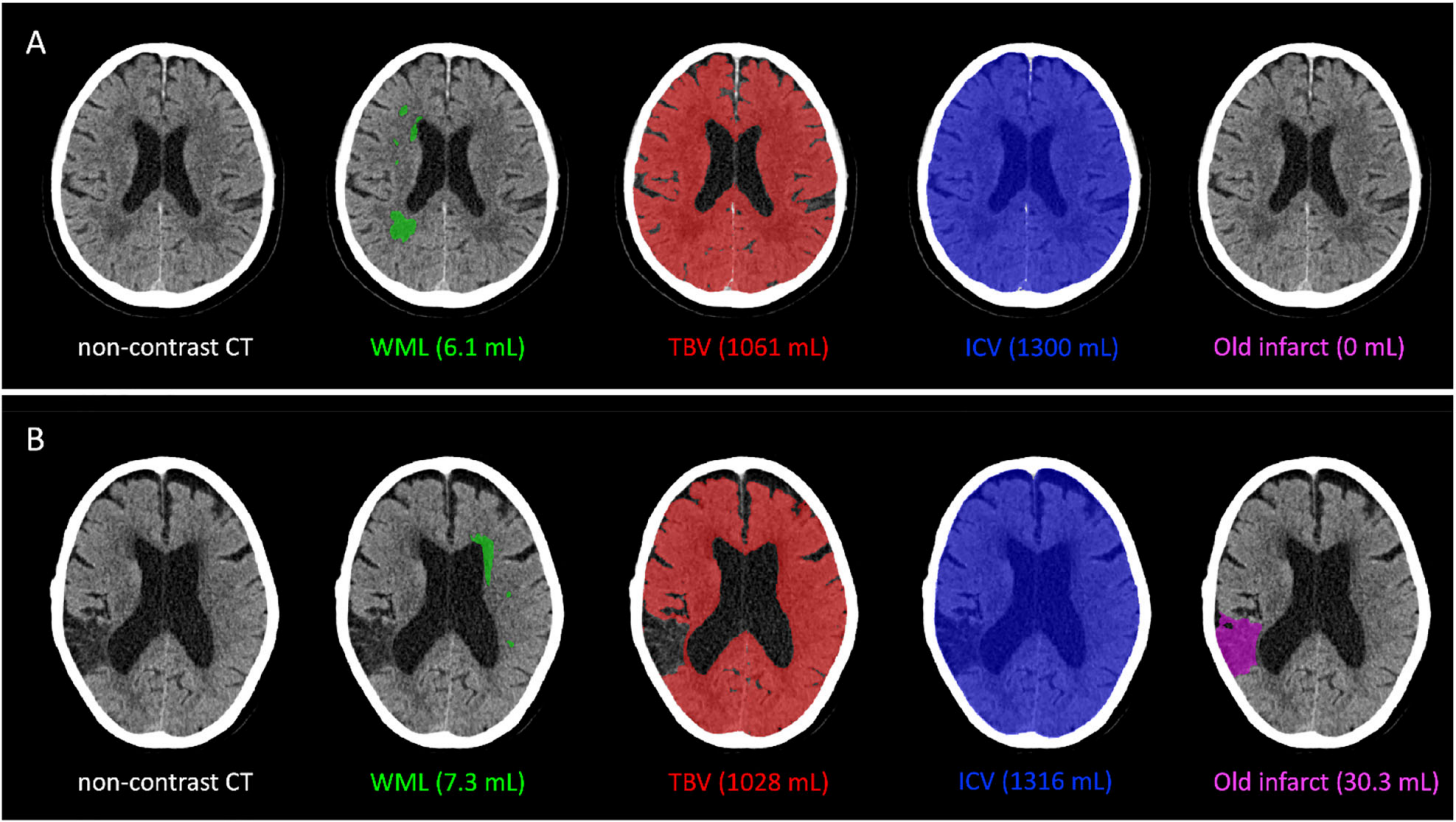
Examples of segmentations on baseline non-contrast CT in two individual patients. A Patient with a left M1 middle cerebral artery occlusion. B Patient with a right M1 middle cerebral artery occlusion. White matter lesion (WML) volume in the asymptomatic hemisphere is shown in green, total brain volume (TBV) is shown in red, and intracranial volume (ICV) is shown in blue. Brain atrophy was determined as the proportion of TBV in relation to ICV expressed as the brain parenchymal fraction (BPF, in %). Old infarct volume shown in magenta
